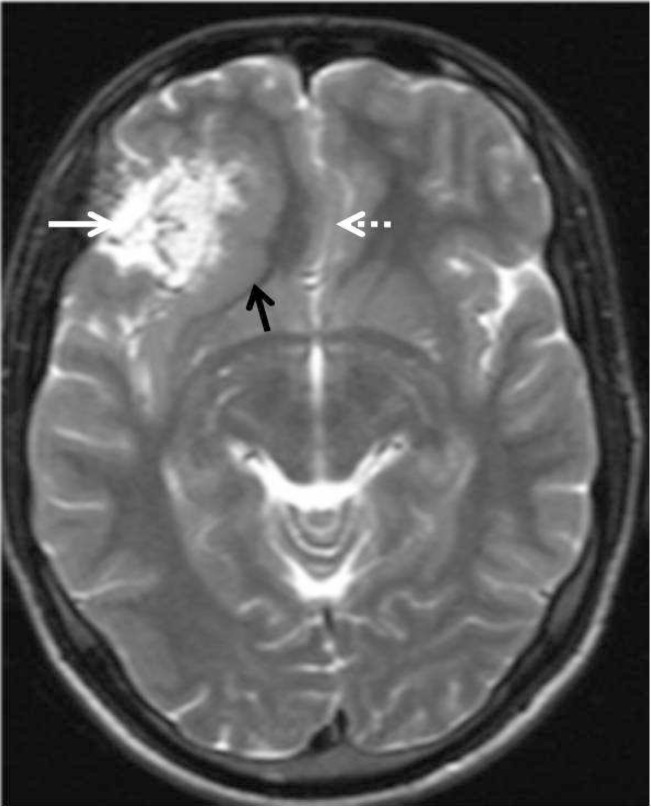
FSE T2-weighted axial image shows hyperintense lesion with flow voids in right sylvian fissure (white arrow) located within a thickened layer of infolding cortex (black arrow). Subfalcine herniation is also seen (dashed white arrow).
